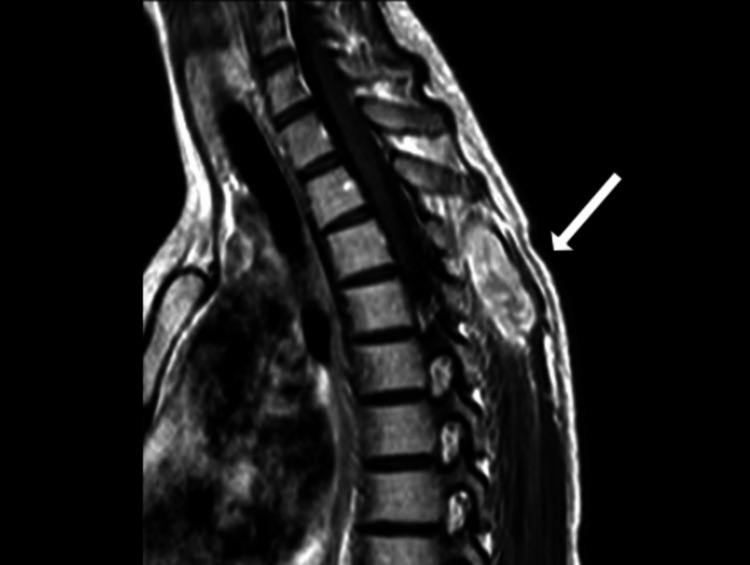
Sagittal T2-weighted MRI image finding of the mass that had grown to 4.1 x 1.7 x 1.5 cm in height, diameter, and width, respectively. The white arrow points to the mass.
